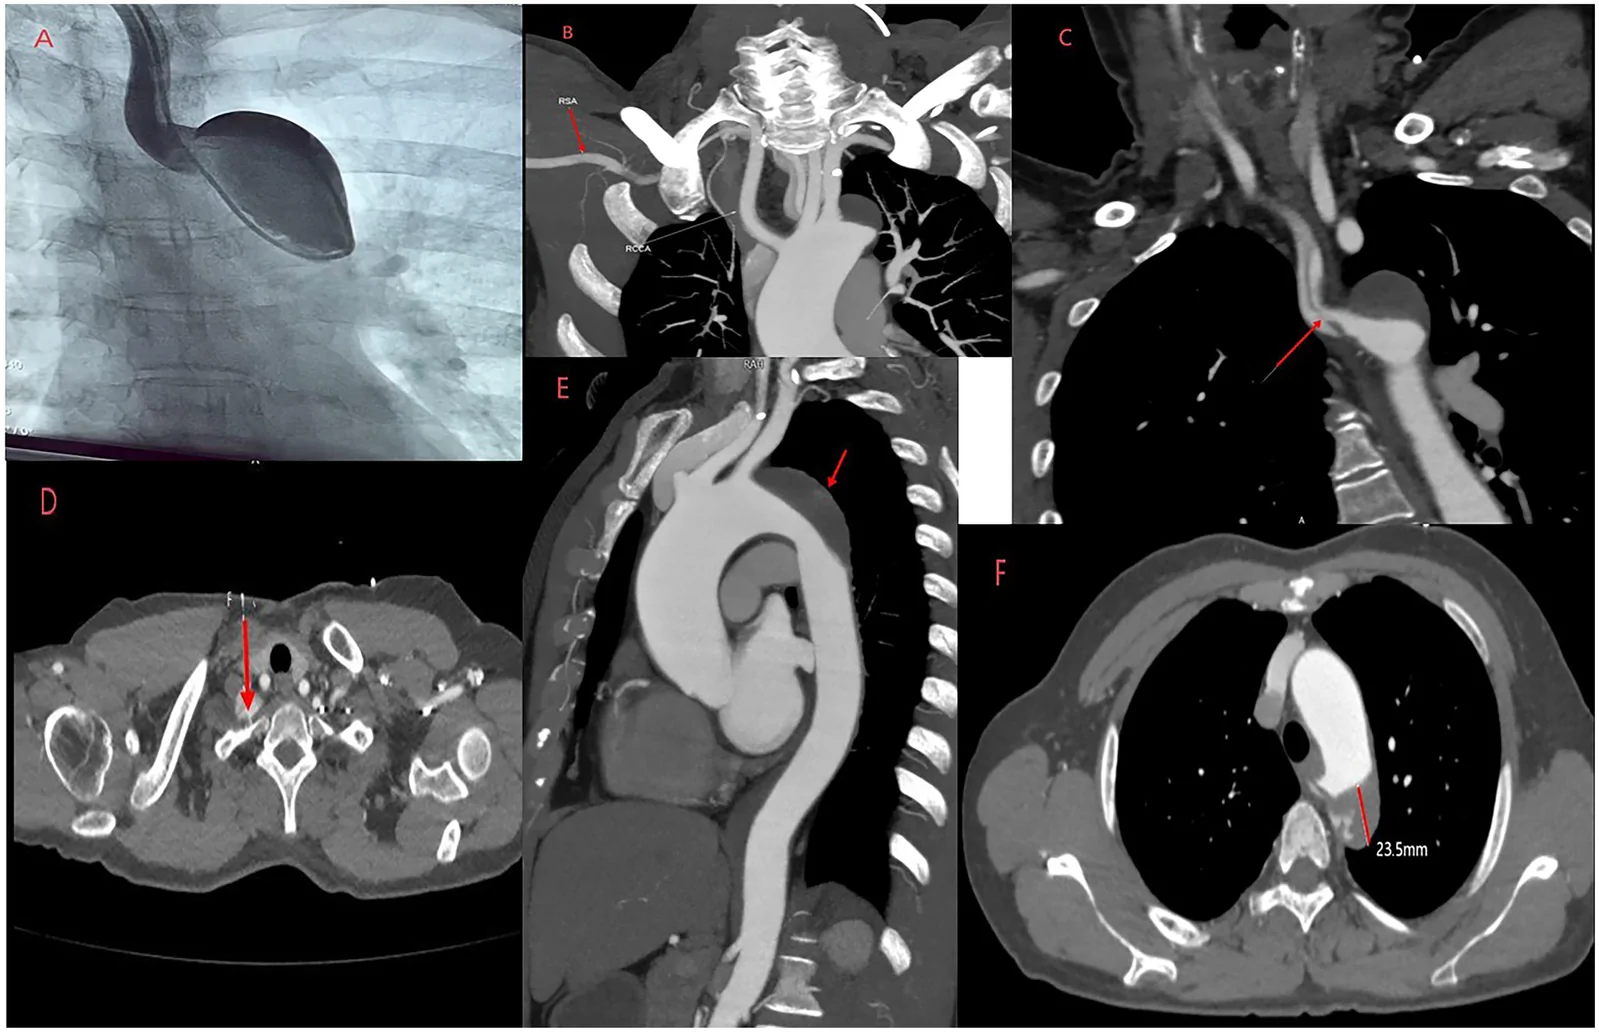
(A) CAG shows contrast filling in the false lumen of the distal aortic arch, descending aorta, and brachiocephalic trunk, confirming the diagnosis of aortic dissection. (B) Whole-aorta CTA identifies an aberrant right subclavian artery coursing posterior to the esophagus toward the right axilla (arrow). (C) CTA demonstrates a dissection lesion (arrow) extending from the proximal right axillary artery to the entire aberrant right subclavian artery. (D) A 5.6-mm intimal tear (arrow) is detected at the proximal segment of the aberrant right subclavian artery on CTA. (E,F) CTA measurements show that the total length of the aortic dissection is approximately 70 mm. The false lumen achieves a maximum width of 23.5 mm (arrow), with predominant thrombosis formation inside the lumen.
